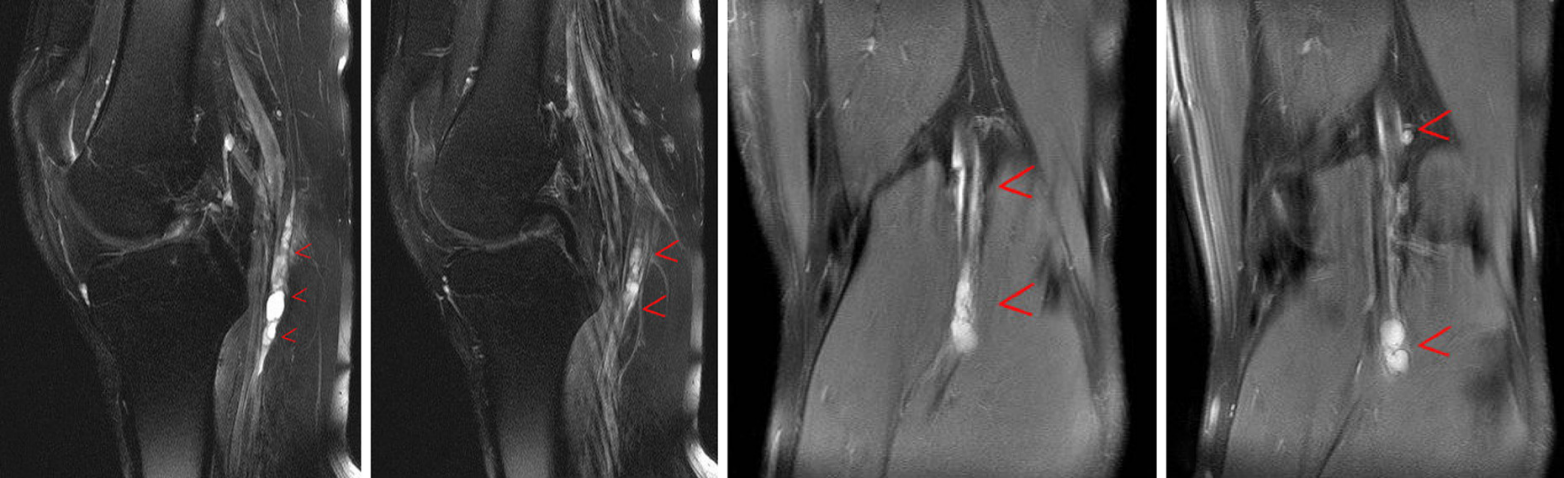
Sagittal and coronal MRI images of the left knee demonstrate a 9.2-cm multilobulated lesion with a vertical orientation in the vicinity of the proximal tibial nerve commencing at the popliteal fossa as highlighted by the red arrows (*T*_2 _turbo spin echo fat-saturated images).
